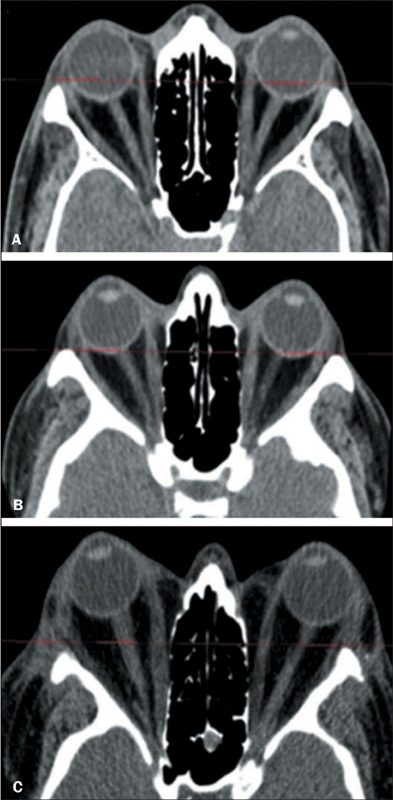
CT scan of orbits in the axial plane: degree of proptosis.
